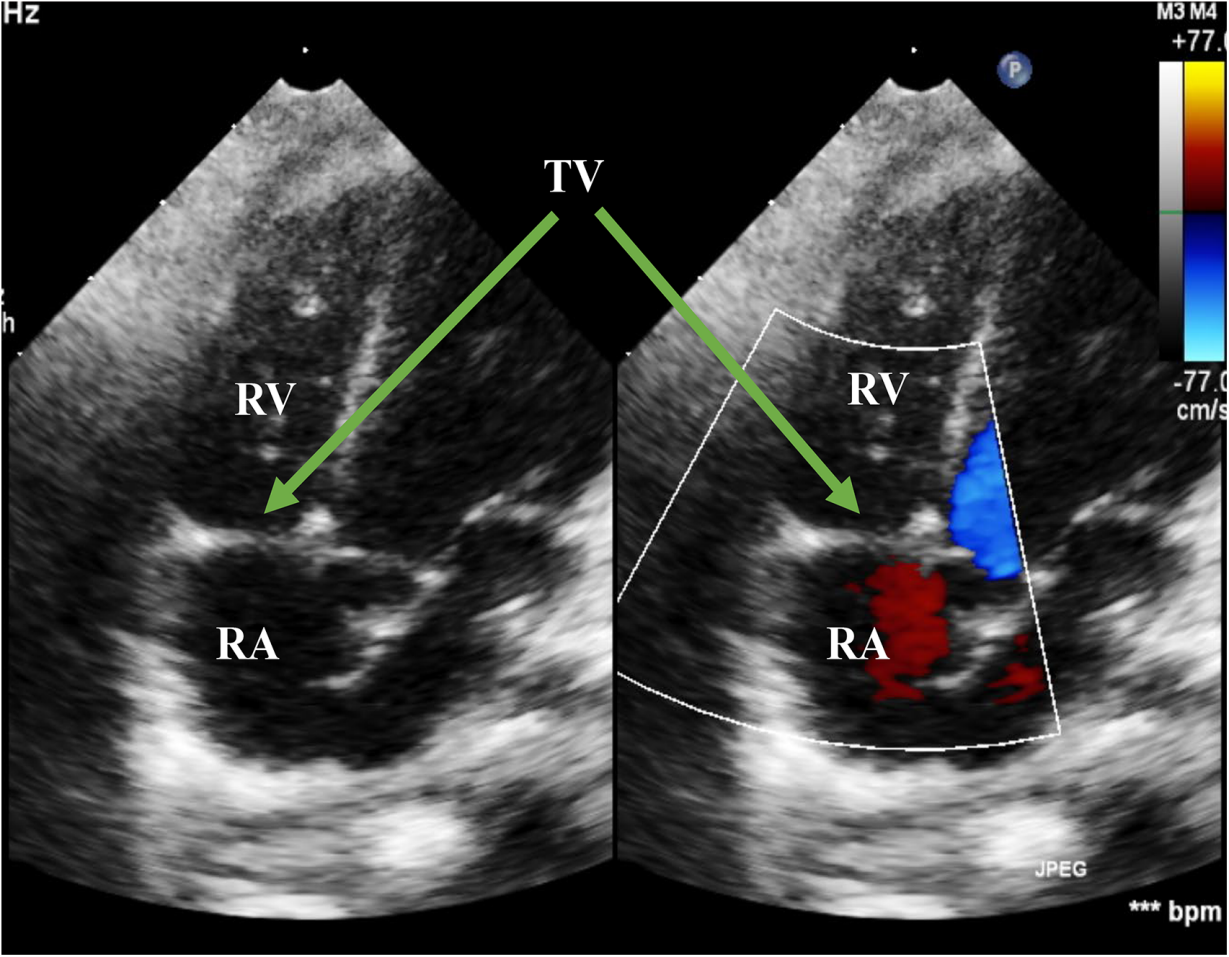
Echo at the latest visit in the A4C view. The figure showed normal movement of the anterior leaflet of the TV in the systolic phase. RA, right atrium; RV, right ventricle; TV, tricuspid valve; A4C, apical four-chamber.
